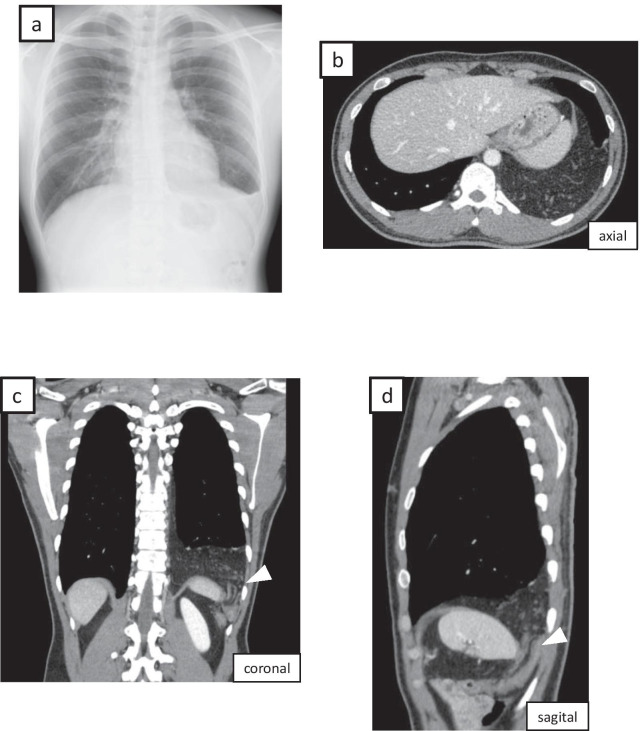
Preoperative diagnostic imaging. a Chest radiography showing an abnormal shadow of the left diaphragm. b Axial computed tomography (CT) showing herniation of omentum into the left thoracic cavity. Coronal (c) and sagittal (d) CT showing omental herniation through the orifice (arrowhead) at the posterolateral diaphragm
